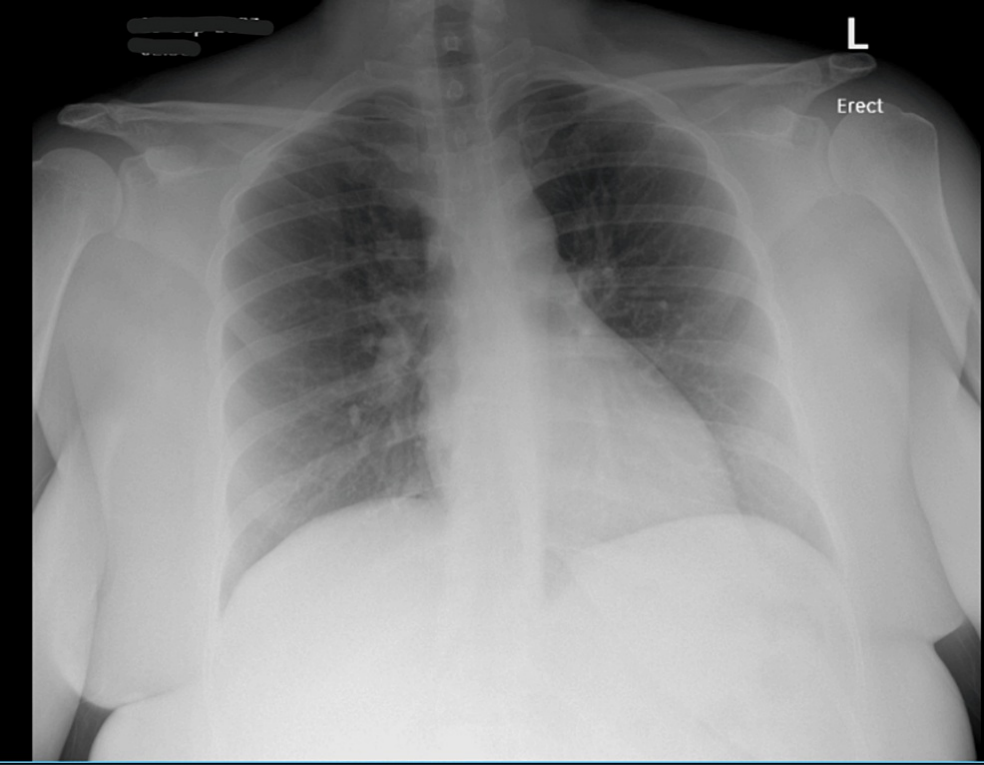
Admission chest X-ray anteroposterior view The chest radiograph on admission shows a normal cardiac silhouette
